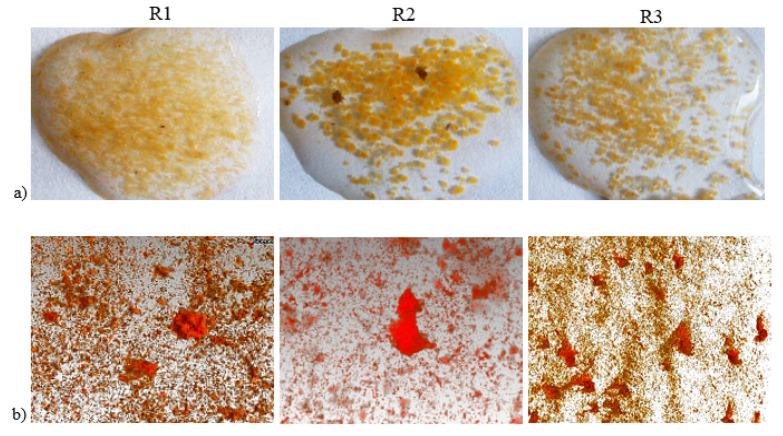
Characteristic biomass: photographs (a) and X-ray tomography images (b) of granules after 89 days of granulation.
